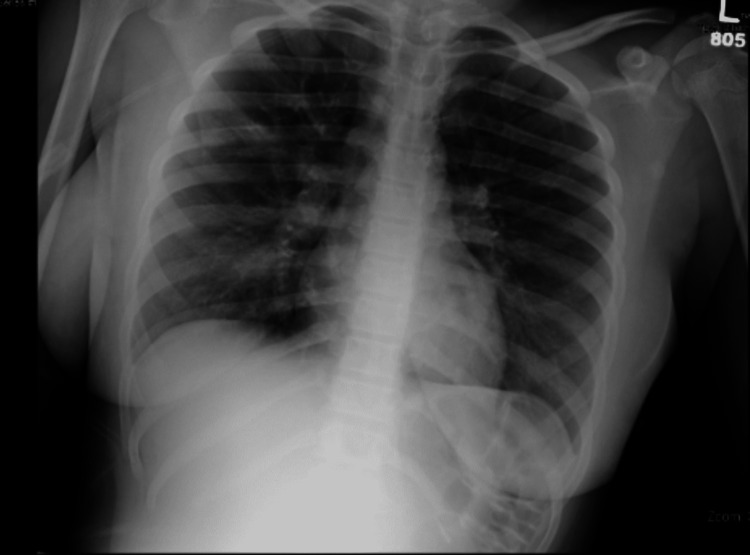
Chest X-ray demonstrating no acute intra-thoracic process.
